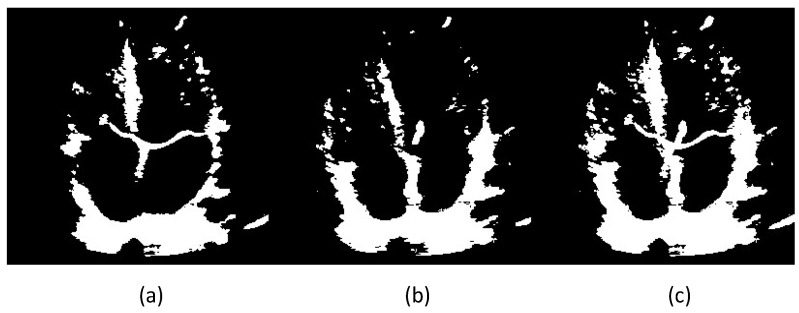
Two frames of a heart’s ultrasound video after binarizing, (a,b) are the two frames whose centroids are farthest from each other, (c) is obtained by combining the two, i.e., $B_{x}+B_{y}$.
